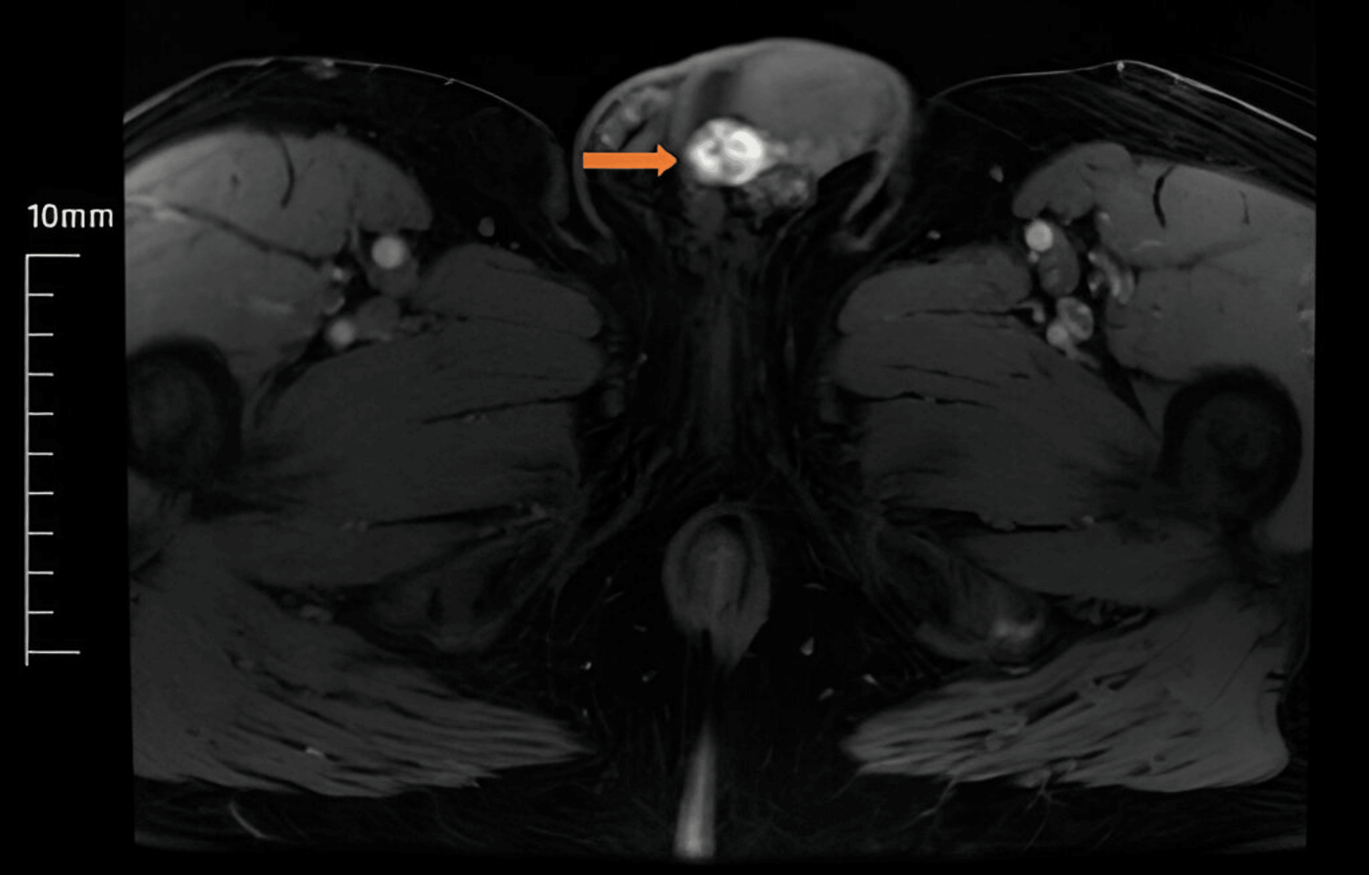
Contrast-enhanced MRI showing a hypervascular lesion (indicated by arrow).
